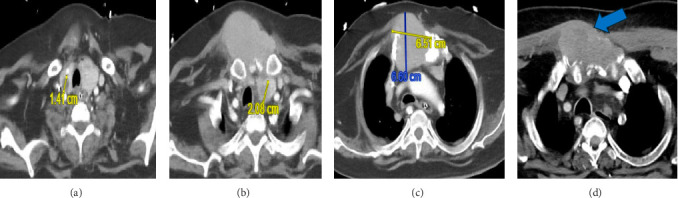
Computed tomography of the chest in axial view showed thyroid enlargement with right thyroid nodule (A) and left thyroid nodule (B) and superior mediastinal mass (C) and subcutaneous extension (blue arrow) and destruction of manubrium sternum (D).
